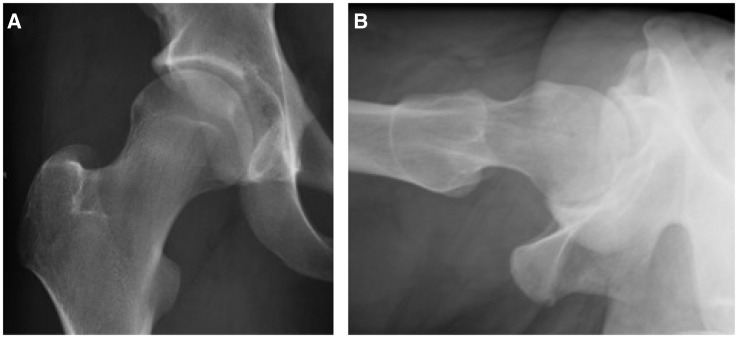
AP (A) and cross-table lateral (B) radiographs of a young athlete with a prominence at the head–neck junction.
